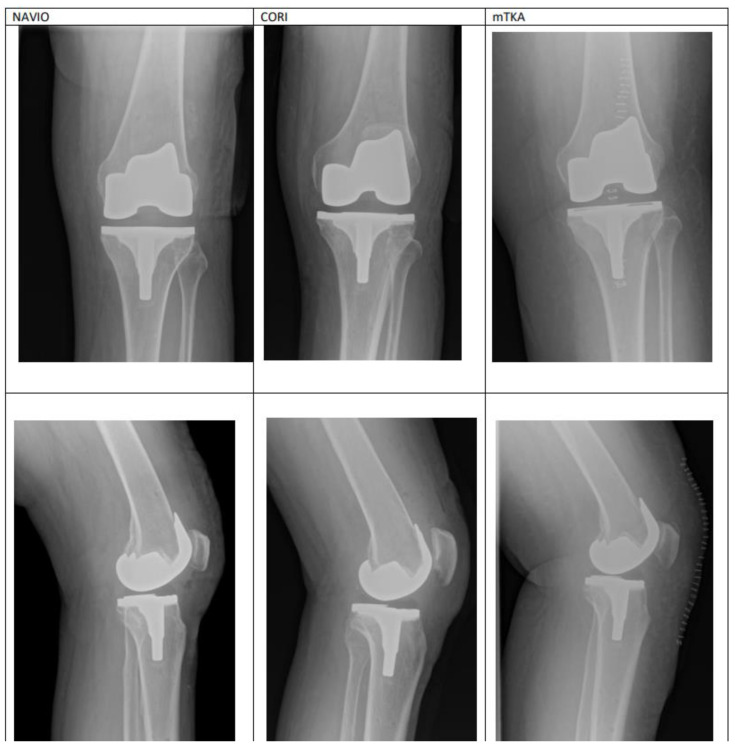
Control entitled X-rays from the day following each procedure: NAVIO and CORI ra-TKA, and mTKA.
